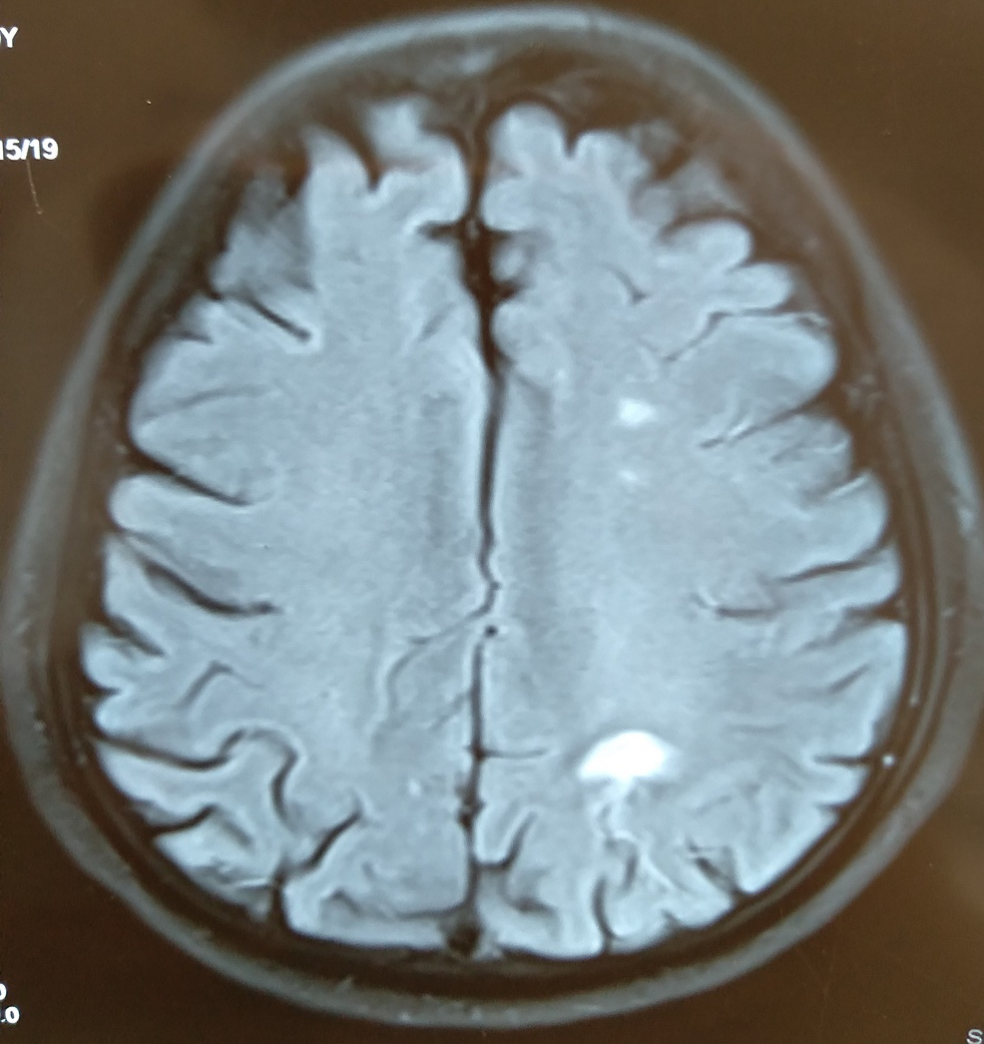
MRI of the brain with DWI The image shows an acute infarct in the border zone of the carotid and vertebral artery region in the left parieto-occipital region DWI: diffusion-weighted imaging; MRI: magnetic resonance imaging
